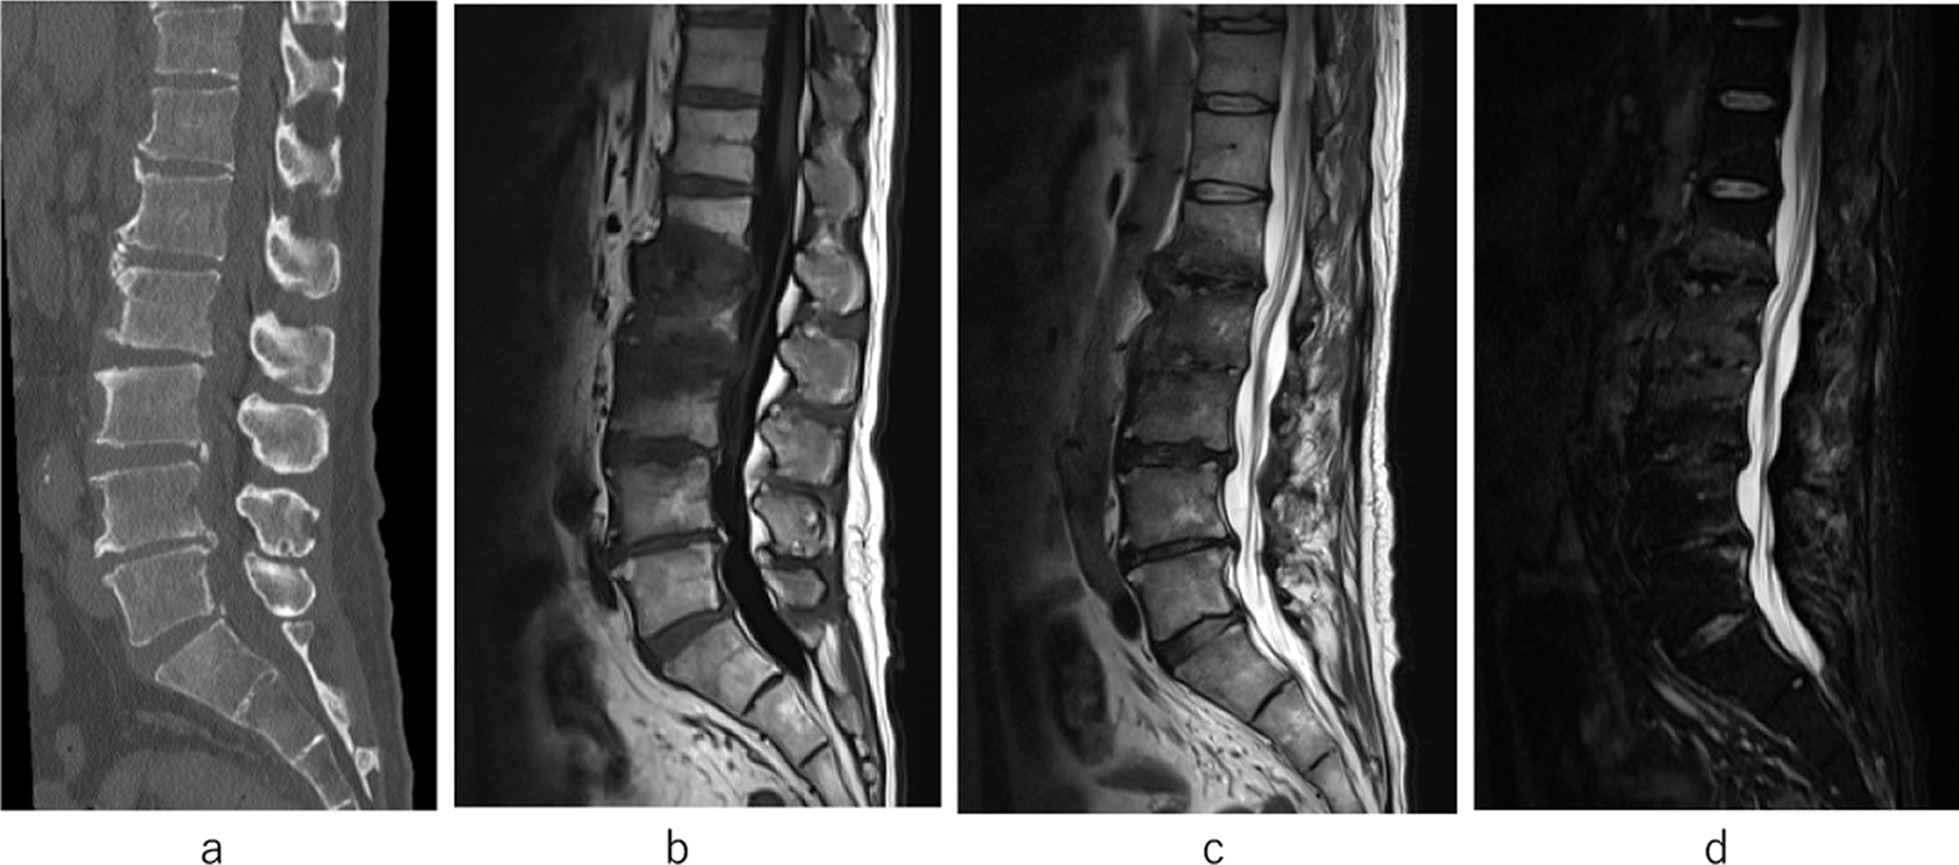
At the first admission. Endplate appeared normal on computed tomography (a). Low signal changes in the L1-2 and L2-3 disc spaces are shown on T1-weighted magnetic resonance images (b) and T2-weighted MR images (c). There was no change on short tau image recovery in MR imaging (d)
